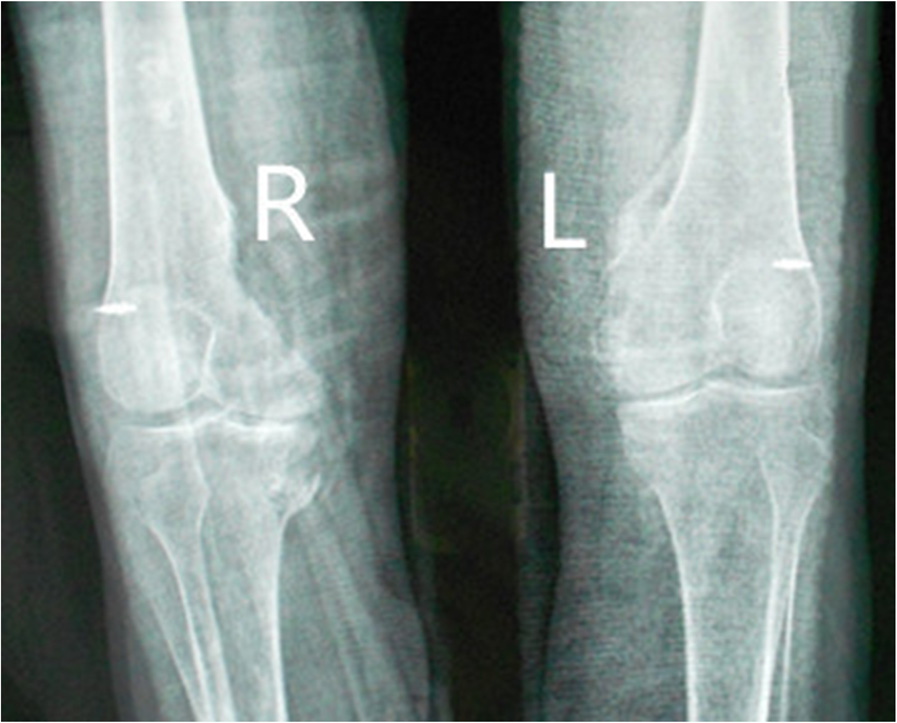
Postoperative radiographs of anterioposterior radiographs of knees shows no loosening of rivets and no recurrence 13 moths after the excision of the ossific mass.
